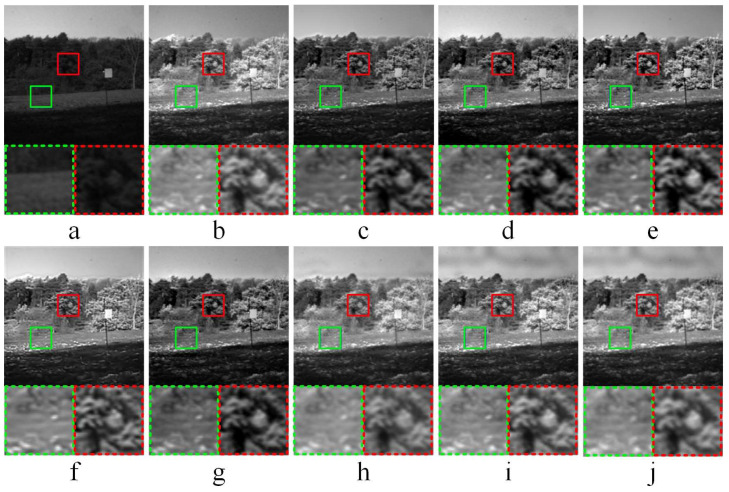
Infrared-visible image fusion comparative experiments—2. (a,b) are source images, (c–j) are the fused results of ASR, CNN, CT, KIM, MST-SR, NSCT-PCNN, NSCT-PC, and the proposed method, respectively. At the bottom of each image, two areas marked in green and red dashed line frames correspond to the magnified areas encompassed in green and red frames, respectively.
